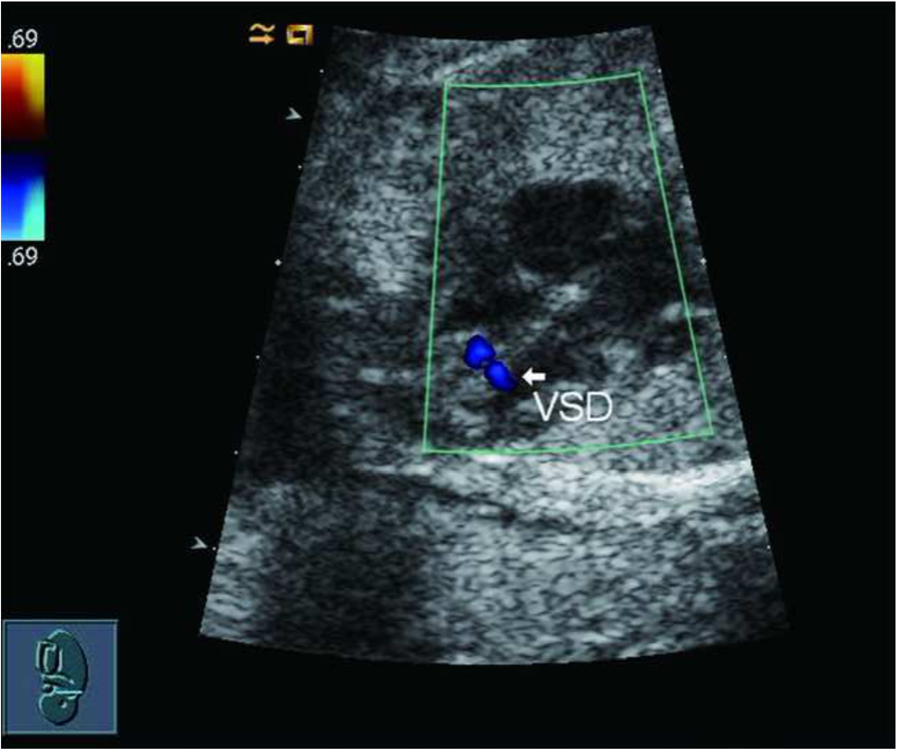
Color Doppler echocardiographic examination of small VSD
